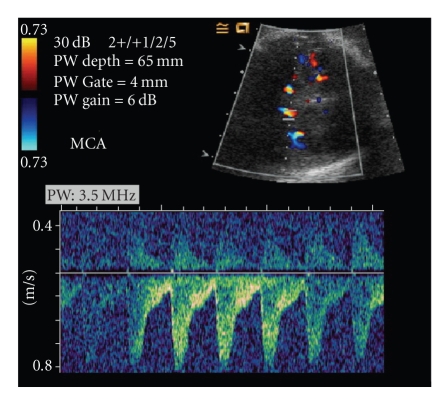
Doppler peak systolic velocity in the fetal middle cerebral artery showing severe fetal anemia.
